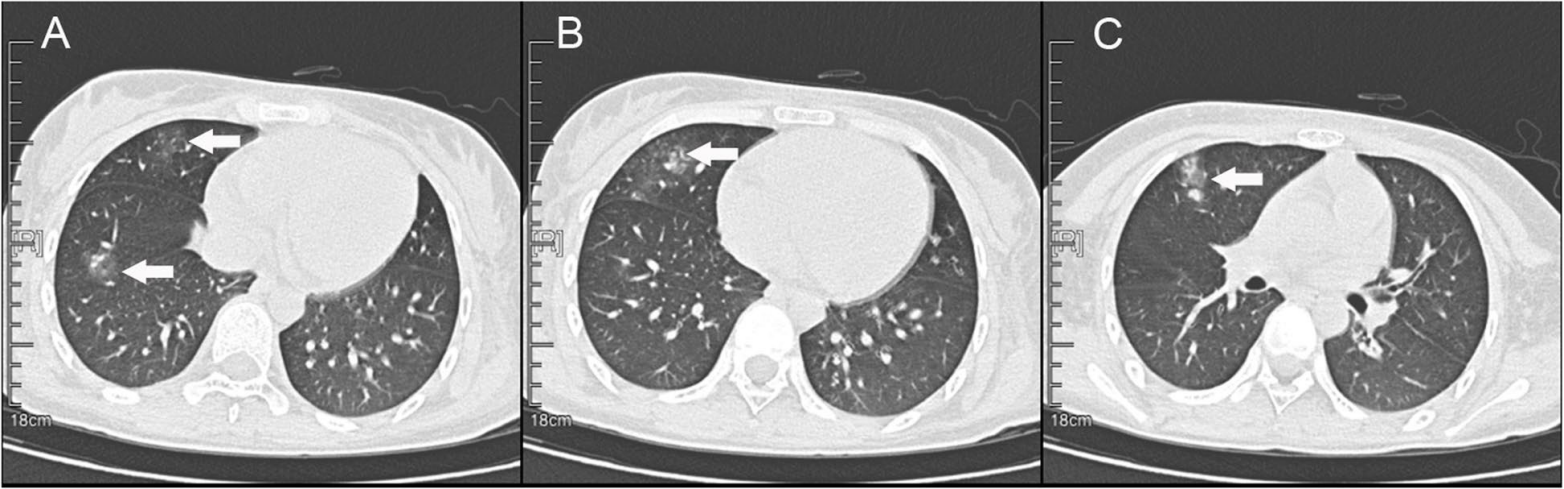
Initial chest CT of the patient. Chest CT images at the onset of the patient’s seizure show pneumonia in the right lung (A-C, white arrow)
